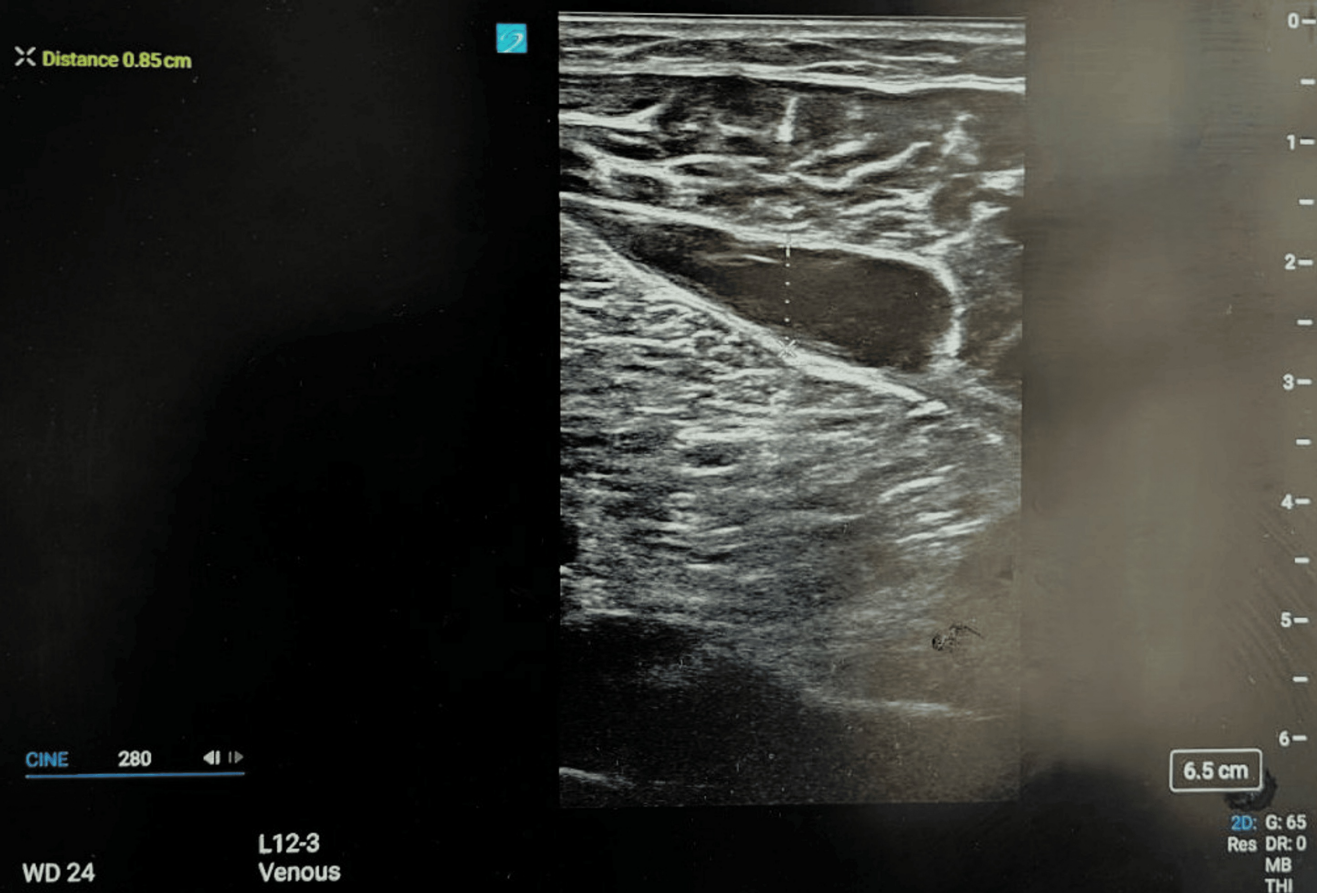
POCUS image showing a well-defined intramuscular hematoma in the right calf POCUS, point-of-care ultrasound
